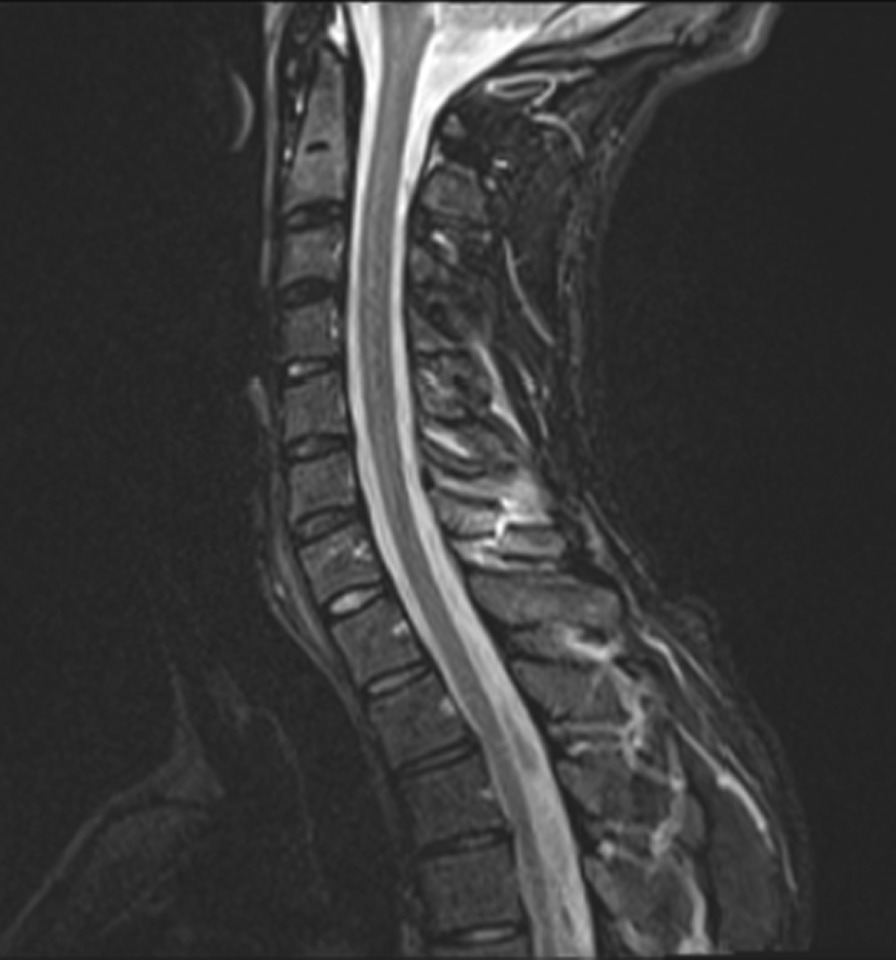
Lateral magnetic resonance imaging (MRI) turbo inversion recovery magnitude (TIRM) sequence. X-ray, computed tomography and MRI taken from patient folder with the patient’s consent
